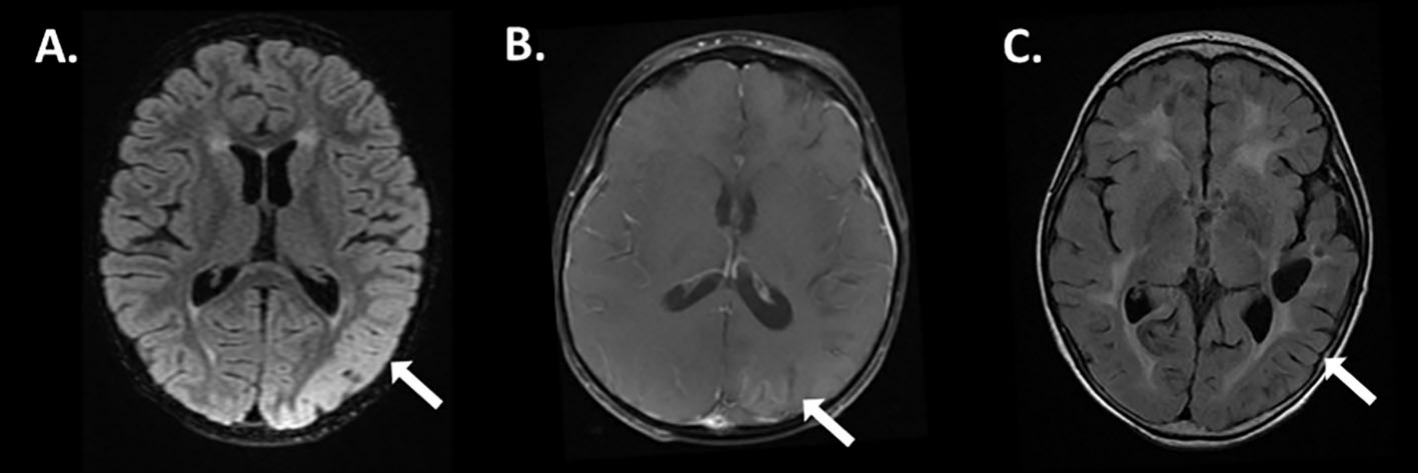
Magnetic resonance imaging features of ici-HHV-6B associated with encephalitis. 3D cube fluid attenuation inversion recovery (FLAIR) sequences at diagnosis revealed hyperintensity with gyral edema in the left posterior temporal lobe (A, arrow), associated with minimal enhancement on post-T1 gadolinium sequences (B, arrow). Six months after resolution of encephalitis resultant volume loss is appreciated on FLAIR sequences (C, arrow).
